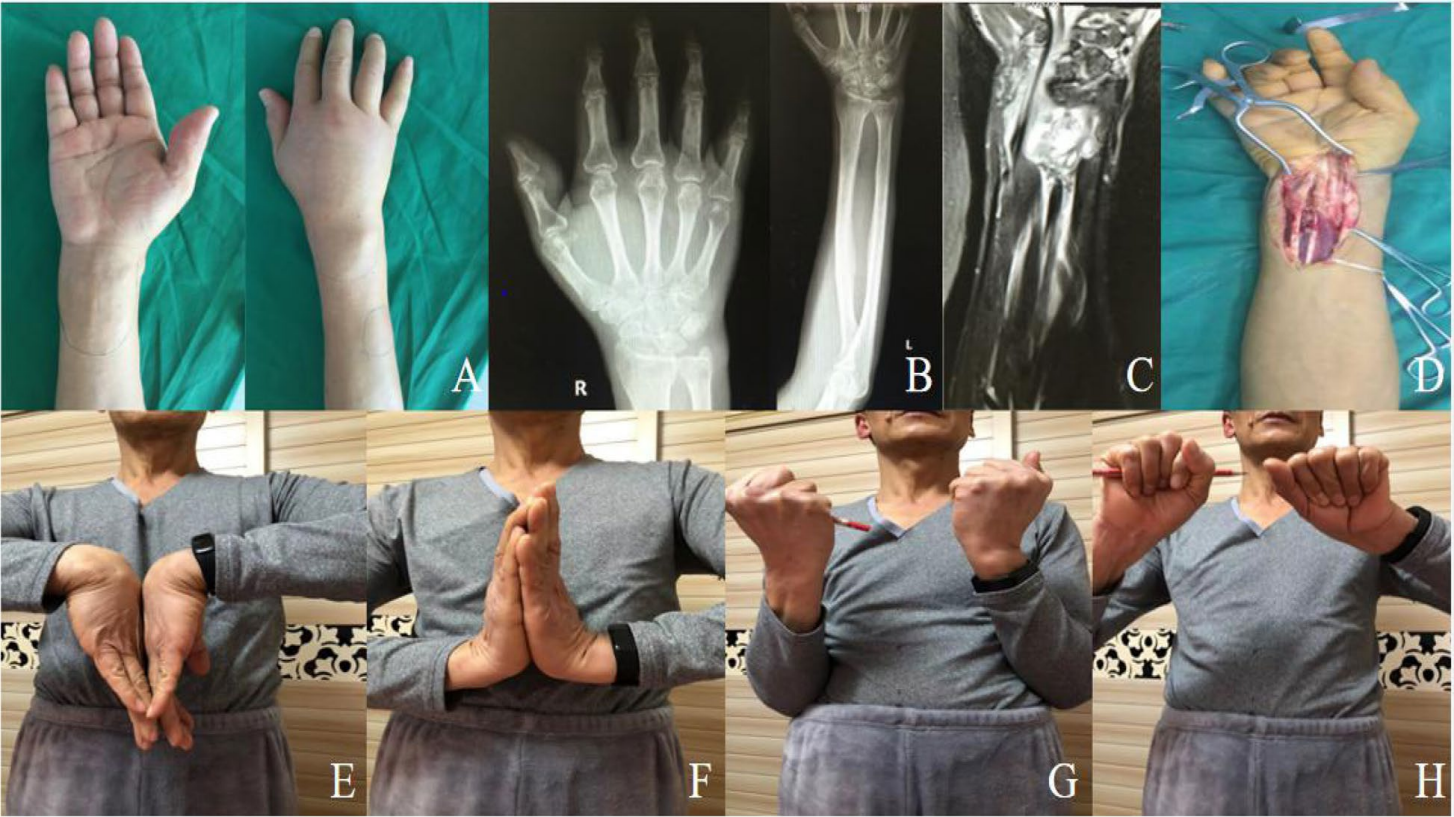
A 52-year-old male patient with Tuberculous tenosynovitis of the wrist. A. Swelling can be observed on the palmar and dorsal side of the right wrist. B. No obvious bone destruction was found on X-ray films of the wrist joint and distal ulnar and radius. C.MRI showed that the synovial lesions of the wrist involve the flexor tendons and the surrounding soft tissue. D. An intraoperative photograph suggested that the degenerated and necrotic synovial tissue attached around the flexor tendons had been completely removed. E–H. The patient's right wrist function recovered well 25.5 months after the operation
